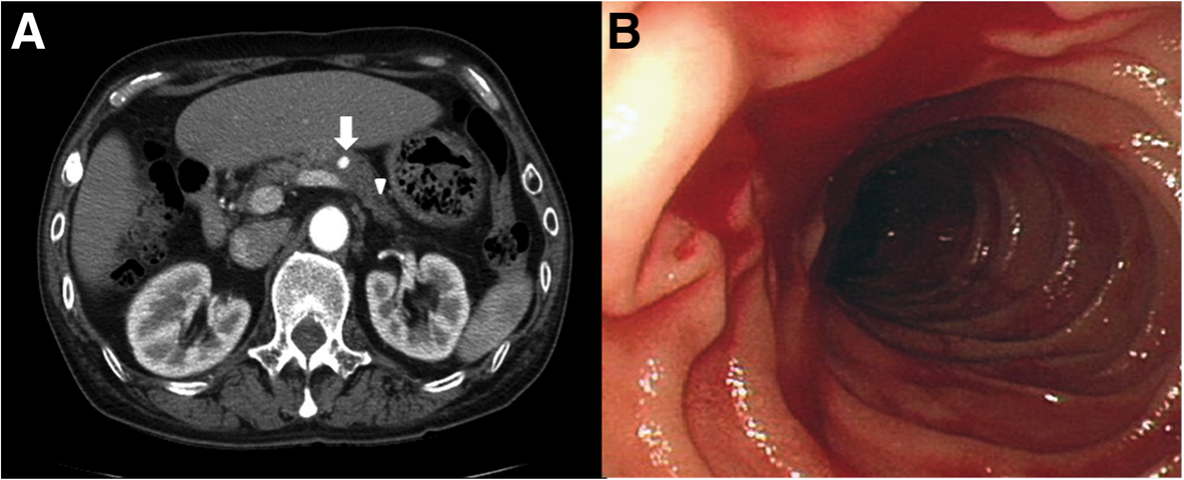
Abdominal CT scan and Endoscopic findings. a 6.0 mm × 10.4 mm saccular aneurysm was evident in the splenic artery (pancreatic segment of the splenic artery) at the body-tail junction of the pancreas (arrow). The aneurysm was out-pouching from the splenic artery perpendicularly down to the pancreas parenchyma. The aneurysm contacted the pancreatic duct, and the pancreatic duct proximal to the aneurysm was not dilated. However, the distal pancreatic duct was slightly dilated (up to 4.0 mm) (arrow head). The surrounding pancreas, especially distal to the aneurysm, was slightly lower in density after contrast enhancement. This finding suggests swelling. b On admission day 9, esophagogastroduodenoscopy showed active hemorrhage from the major papilla
